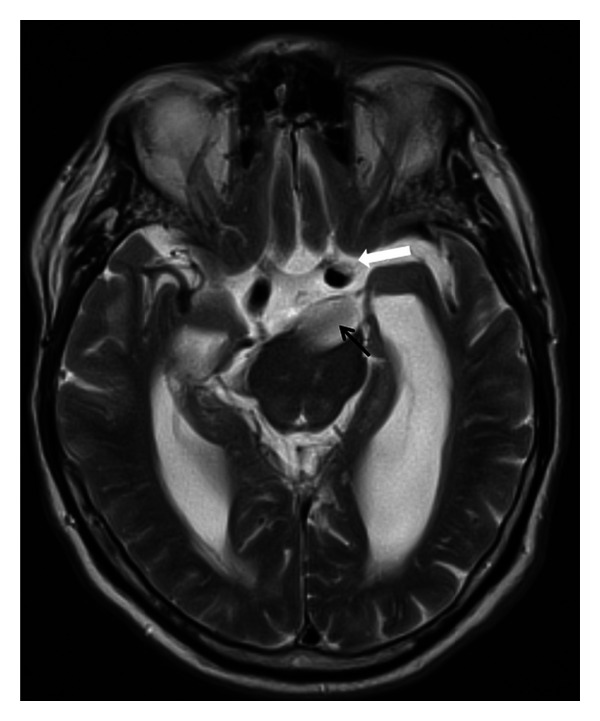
Magnetic resonance imaging (T2-weighted axial view). Ectatic basilar artery trunk compressing on the pons and the retrochiasmal pathway (black arrow depicting lumen of the basilar artery). Note the displaced internal carotid artery adjacent to the left optic nerve (white arrow).
